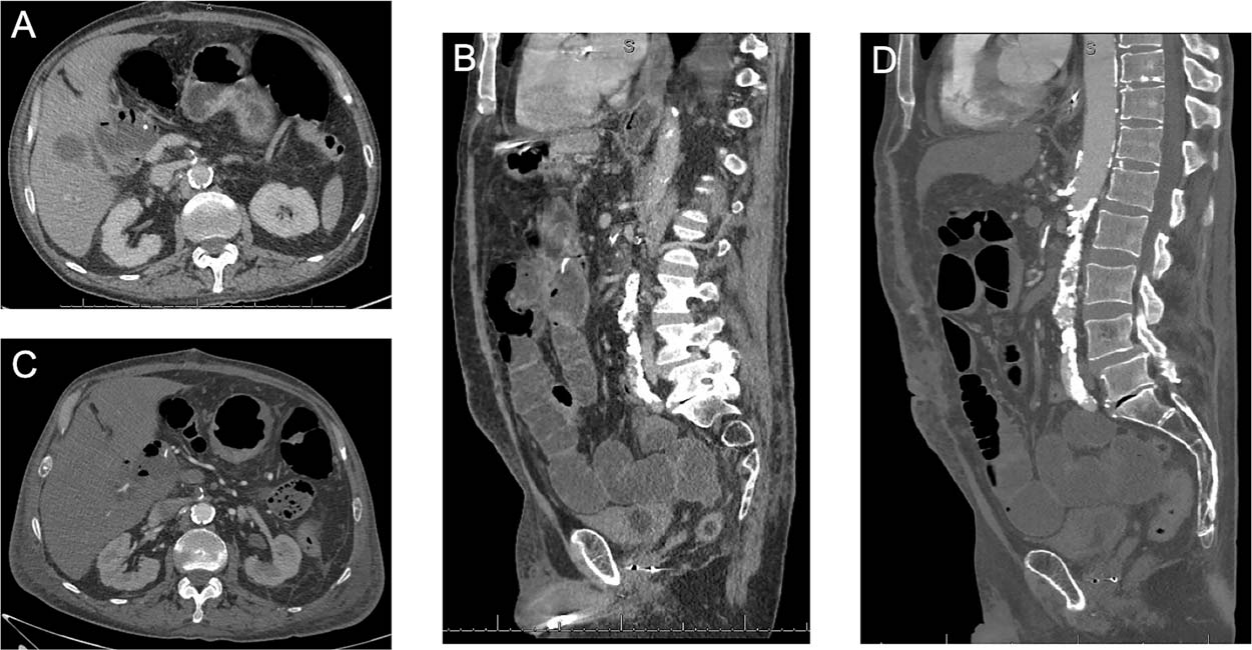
(A–B) Axial and coronal CT images showing the diseased celiac and SMA with flow at POD #10. (C–D) Axial and coronal CT images showing the diseased SMA with a new occlusive thrombus at POD #12.
